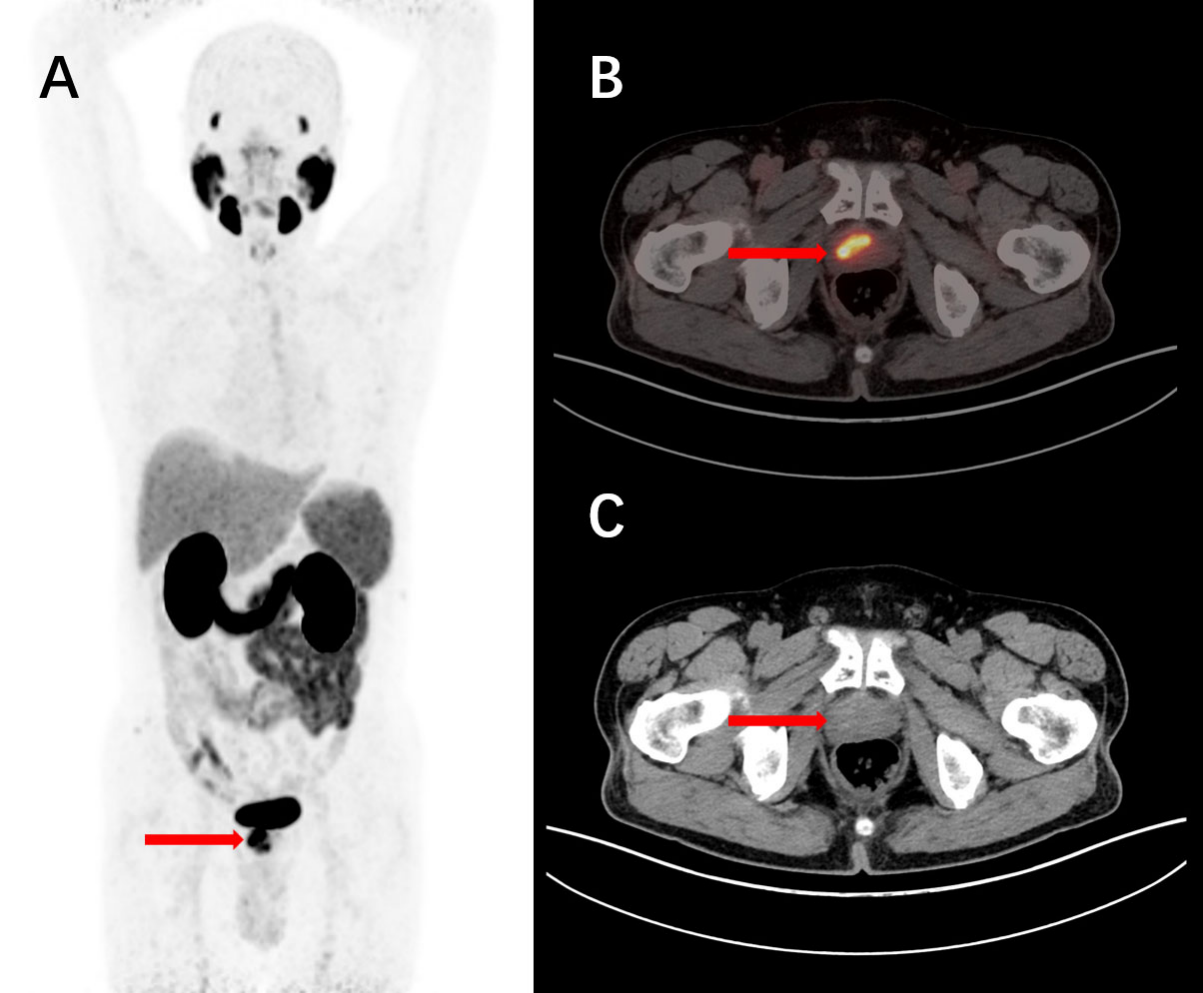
A 76-year-old male, diagnosed with prostate cancer for over 4 years and untreated, underwent intravenous injection of ^68^Ga-PSMA-11 followed by PET/CT tomographic imaging. The maximum intensity projection (MIP) image showed an elevated focal uptake in the nodular structure of the prostate (A). The tomographic images revealed a nodular increased PSMA expression focus in the right portion of the prostate parenchyma, with a slightly decreased density in the corresponding area. Nodular high-density shadows were observed within the parenchyma (B, C). These findings are consistent with the manifestation of prostate cancer.
